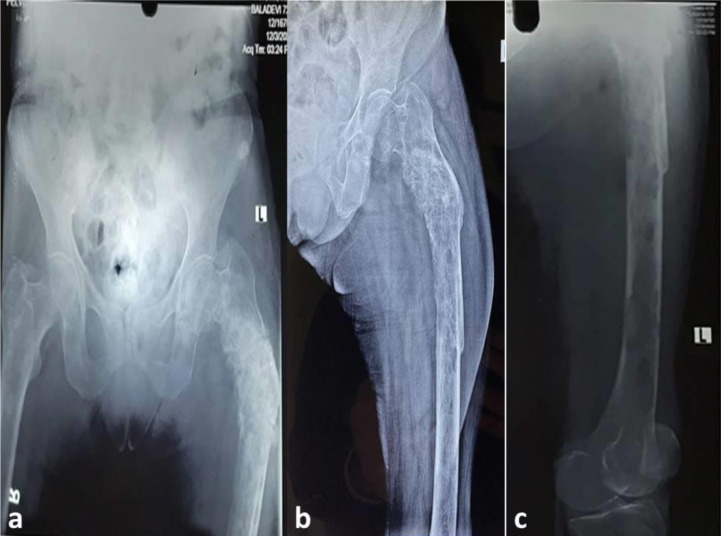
Plain radiographs of the pelvis (a) and left thigh (b, c) show multiple areas of osteolysis in the proximal femur with gross deformation and cortical breach. Numerous moth-eaten and permeative lucencies throughout the proximal and shaft of the femur can be appreciated. There is no apparent periosteal reaction or soft tissue component.
